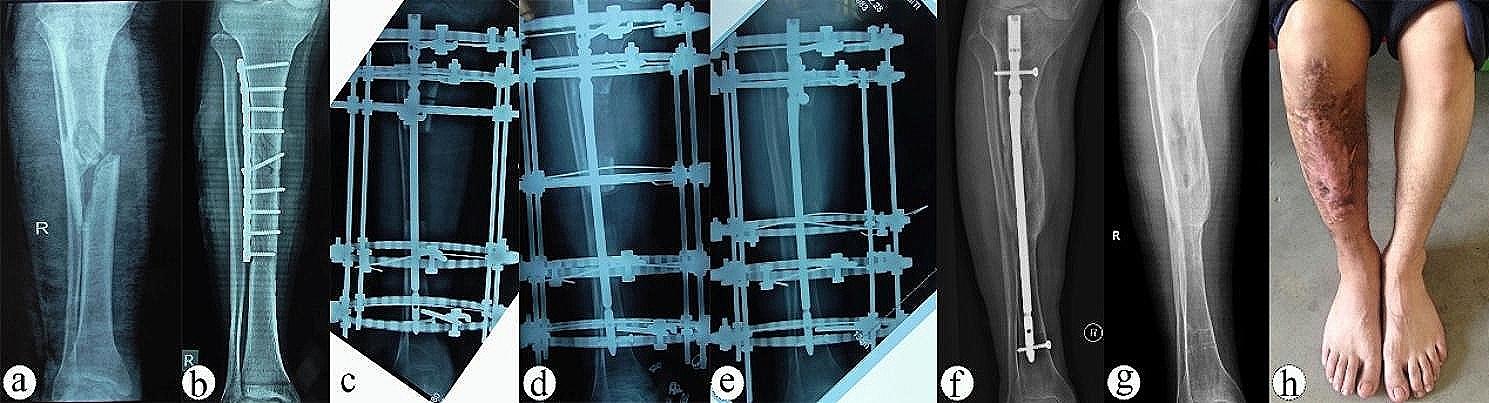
32-year-old male with a right tibial bone defect caused by post-traumatic osteomyelitis was treated by bone transport using EFOIN. a, b) X-ray showed that there was a nonunion of the right tibia after internal fixation. c) The bone defect was approximately 9.8 cm after radical debridement and treated by bone transport using a circular external fixator over an intramedullary nail. d, e) The transport bone segment reached the docking site at 15 postoperative weeks, and the regenerated callus was good without axial deviation. f–h) Satisfactory bone healing and function recovery of the right lower limb was achieved at 12 postoperative months
